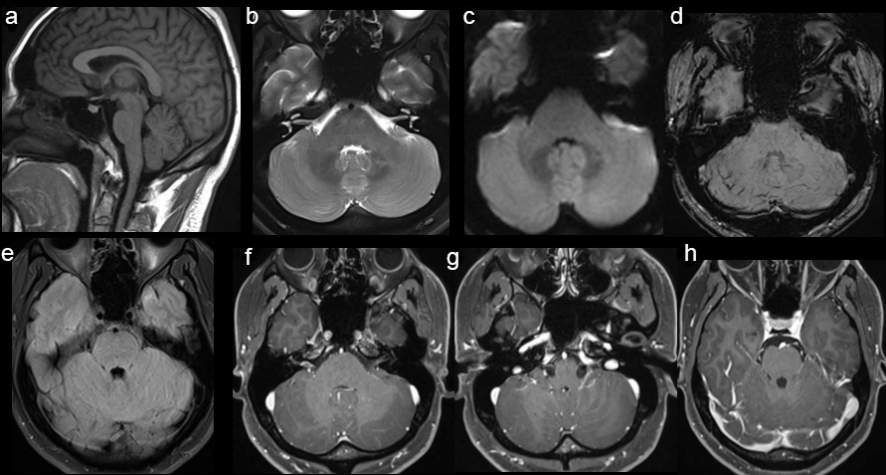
(a) Sagittal MR T1-weighted images, (b) axial T2-weighted images, (c) axial DWI, (d) axial susceptibility-weighted images (SWI) and (e) axial T2-FLAIR MRI continued demonstrating no abnormalities. (f, g and h) Axial *T*
